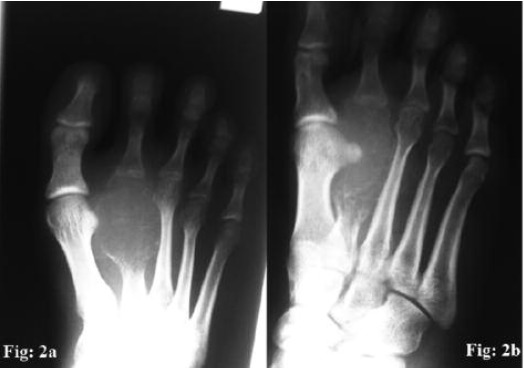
Radiograph of right foot showing extent of tumor on
